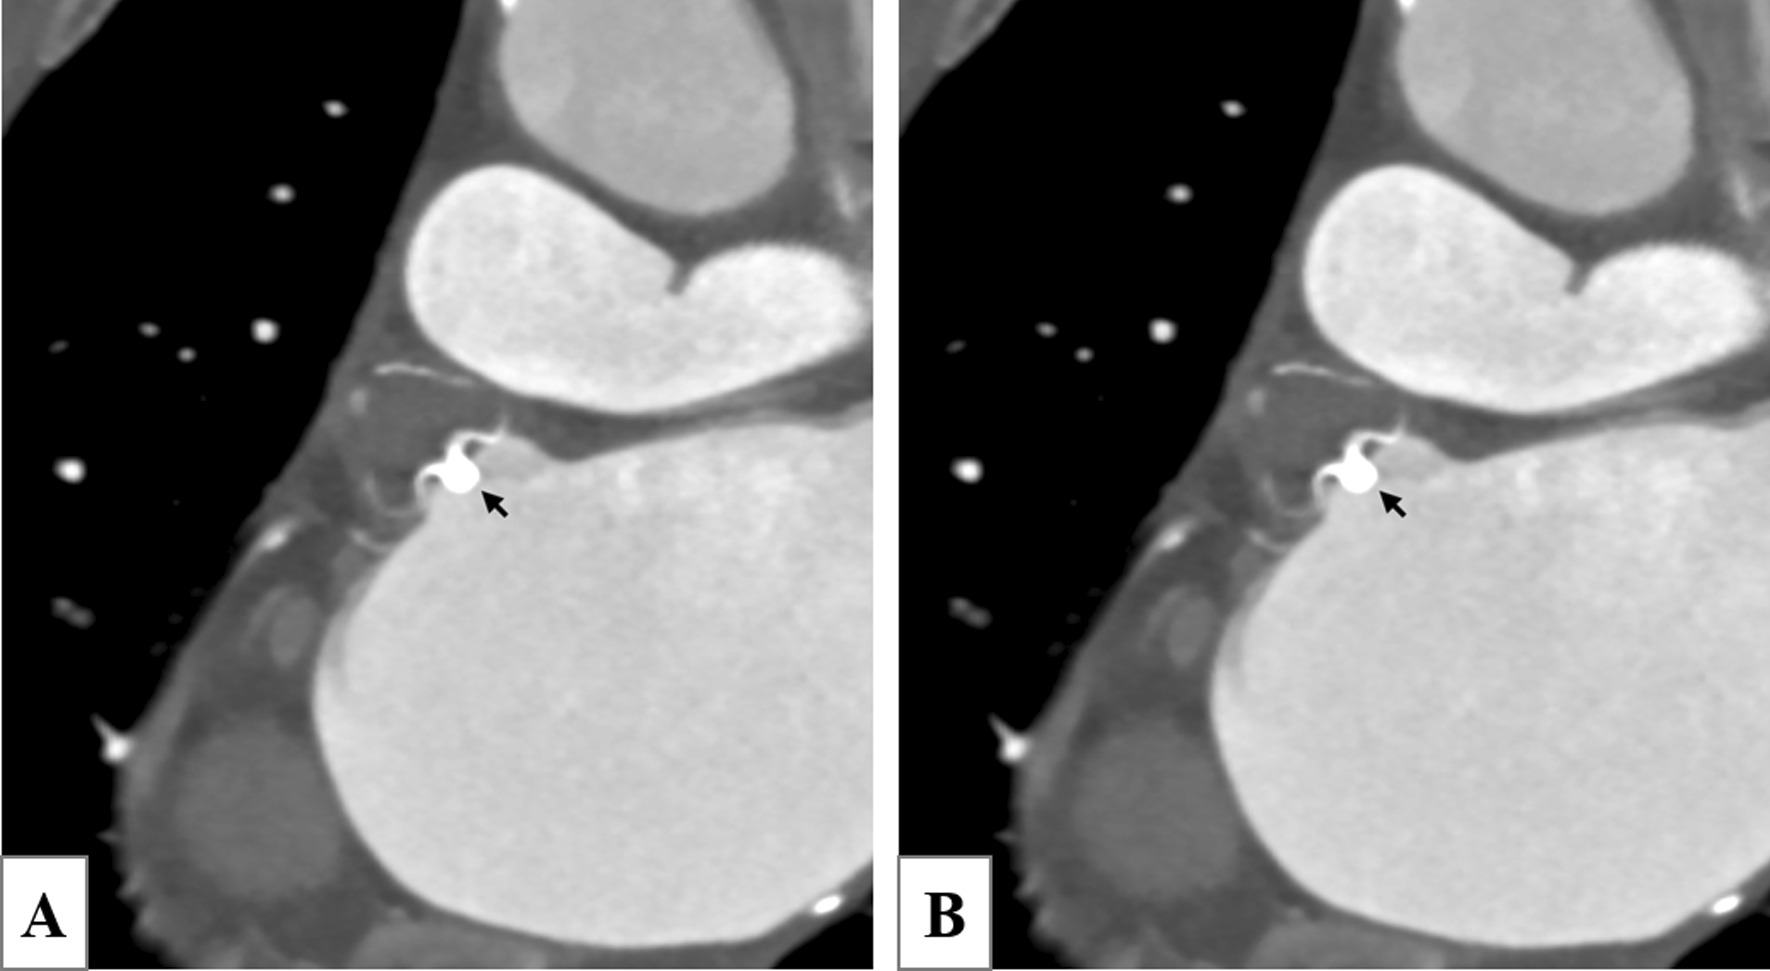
CTA at 6 months shows that the long axis of the sealing device fits well with the long axis of the orifice. There is no contrast agent leakage and no contrast agent filling in the sealing device, indicating that the sealing is successful and the effect is good (A). The arrow shows the short axis of the auricular orifice. CTA shows that the long axis of the sealing device fits well with the anchoring area of the auricular orifice. There is no thrombosis on the surface of the device, and the sealing effect is good (B)
